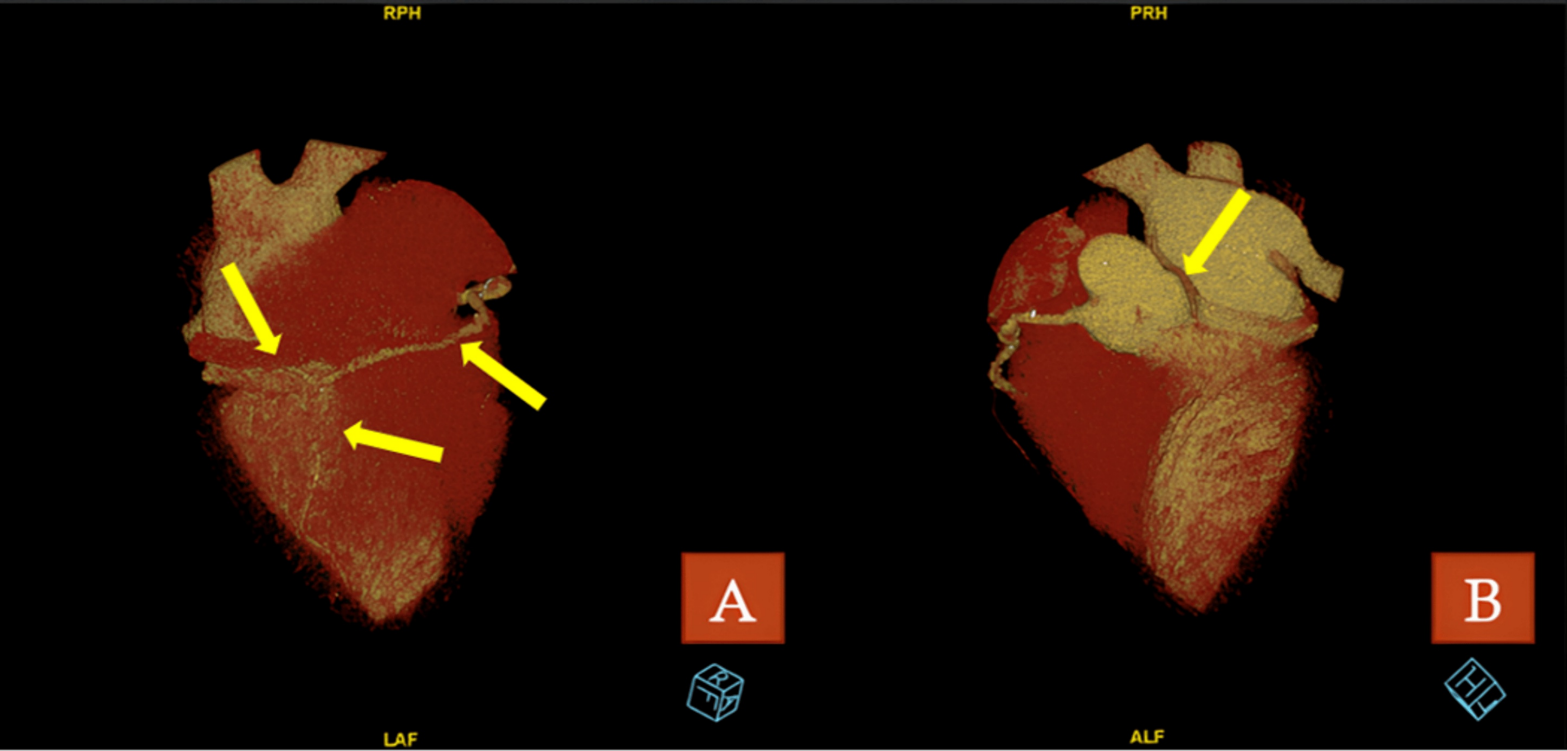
Three-dimensional reconstruction of coronary arteries in CCT angiography. (A) Severe stenoses in PDA and PLVA. (B) Absence of LAD and CX arteries, single RCA. CCT: cardiac computed tomography; LAD: left anterior descending coronary artery; CX: circumflex artery; RCA: right coronary artery; PLVA: posterior left ventricular artery; PDA: posterior descending artery
